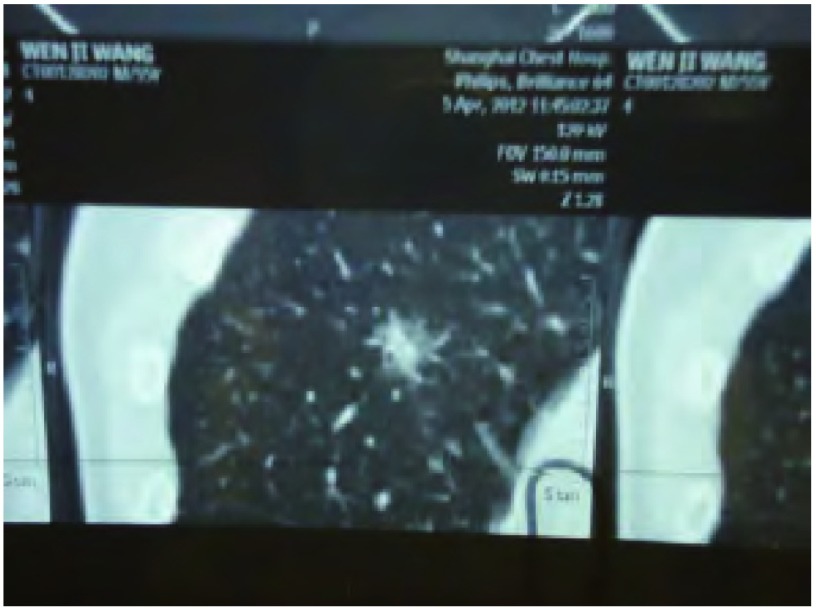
胸部CT病灶 The lesion of CT scan. CT: computed comography.
